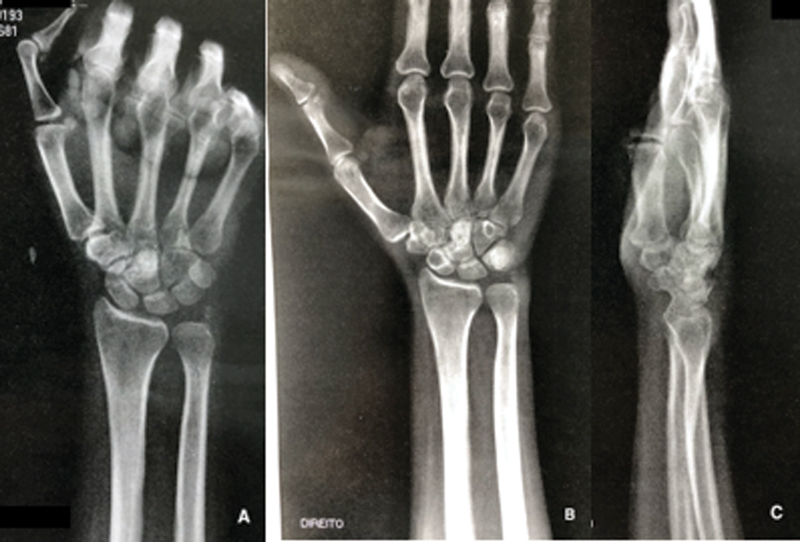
Radiographs of the right wrist eight weeks after surgery. Good integration of the bone graft into the right capitate bone, with no signs of lesion recurrence. Posteroanterior view with clenched fist (
